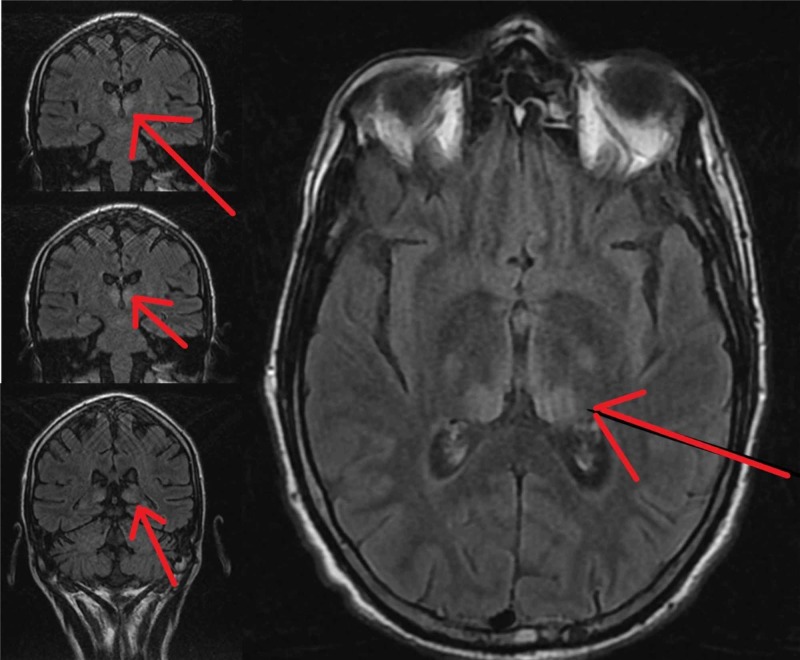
T2 FLAIR MRI of the brain On the T2 FLAIR MRI of the brain it showed bilateral thalamic hyperdensities (red arrows).
